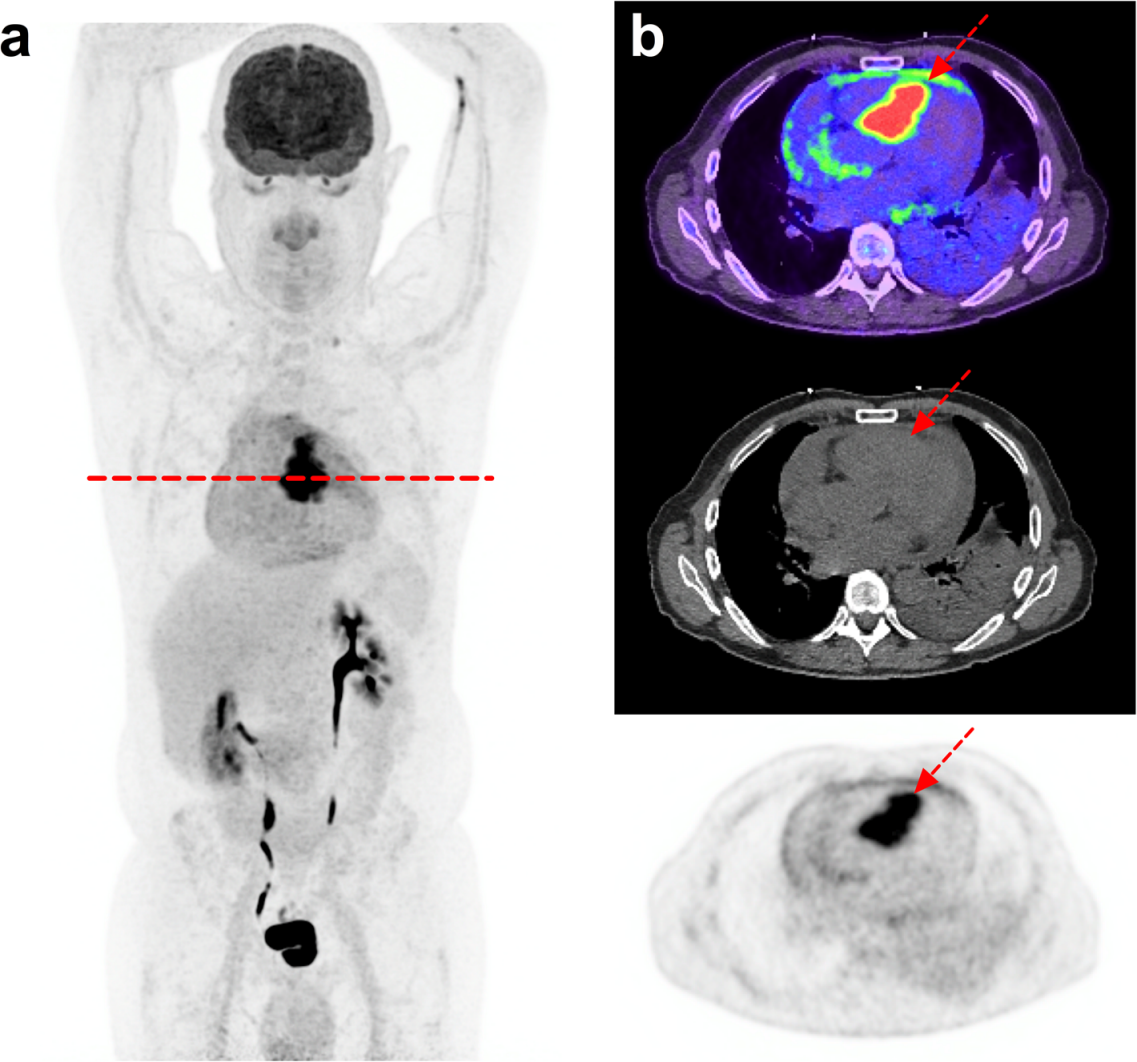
69-year-old male referred for suspicion of myocardial metastasis from melanoma. From left to right, MIP PET (a) and axial fused PET/CT, CT and PET images (b): Prolonged fasting (12 h), carbohydrate-restricted diets, fatty meals, and heparin loading (50 UI/Kg 15 min prior to FDG injection) allowed suppression of physiological myocardial uptake and clear visualization of a septal metastasis and metastatic pericardial effusion
